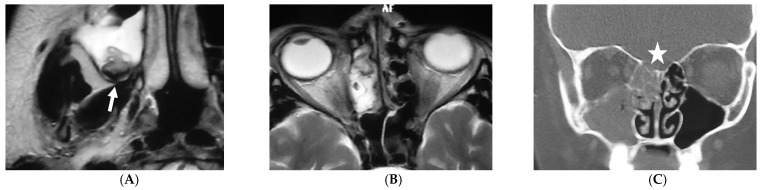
A 7-year-old girl with relapse of acute lymphoblastic leukemia (ALL) and biopsy-proven aspergillosis of paranasal sinuses. The patient has deceased. Axial T2-weighted magnetic resonance images (MRI) (A and B) show abnormal soft tissue filling the ethmoidal cells and in maxillary sinus on the right with T2-hypointensity (straight arrows). Coronal computerized tomography (CT) (C) shows destruction of the sinus walls and the cribriform plate (star) with extension into the anterior cranial fossa. Follow-up images (not shown) showed multiple parenchymal bleedings on the frontal lobes.
